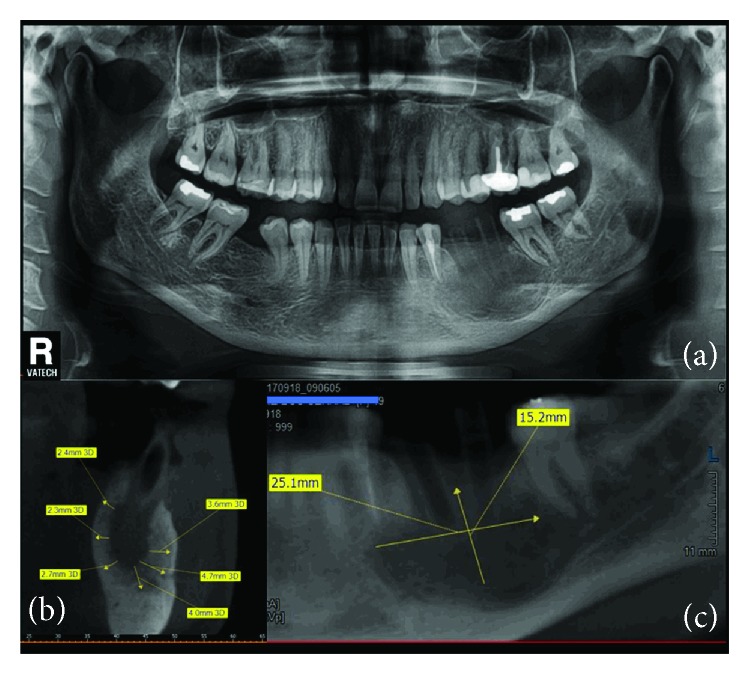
(a) Panoramic radiograph 7 months after decompression, showing a reduction of the volume of the lesion. (b) CBCT of the same time period time, the para-axial cut shows a bone healing toward the center of the lesions with approximately ±4 mm buccally and ±2.4 mm lingually. (c) Sagittal reconstruction of the CBCT shows a volume reduction of the lesion approximately.
